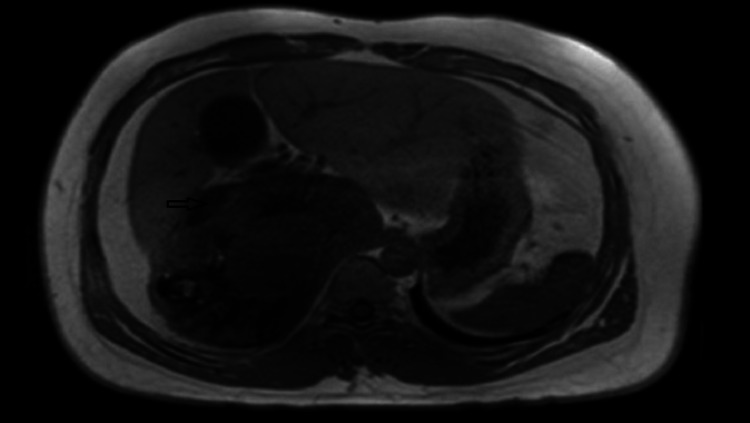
MRI showing a huge right retroperitoneal tumor pushing the IVC (arrow) without invasion. IVC: Inferior vena cava.
